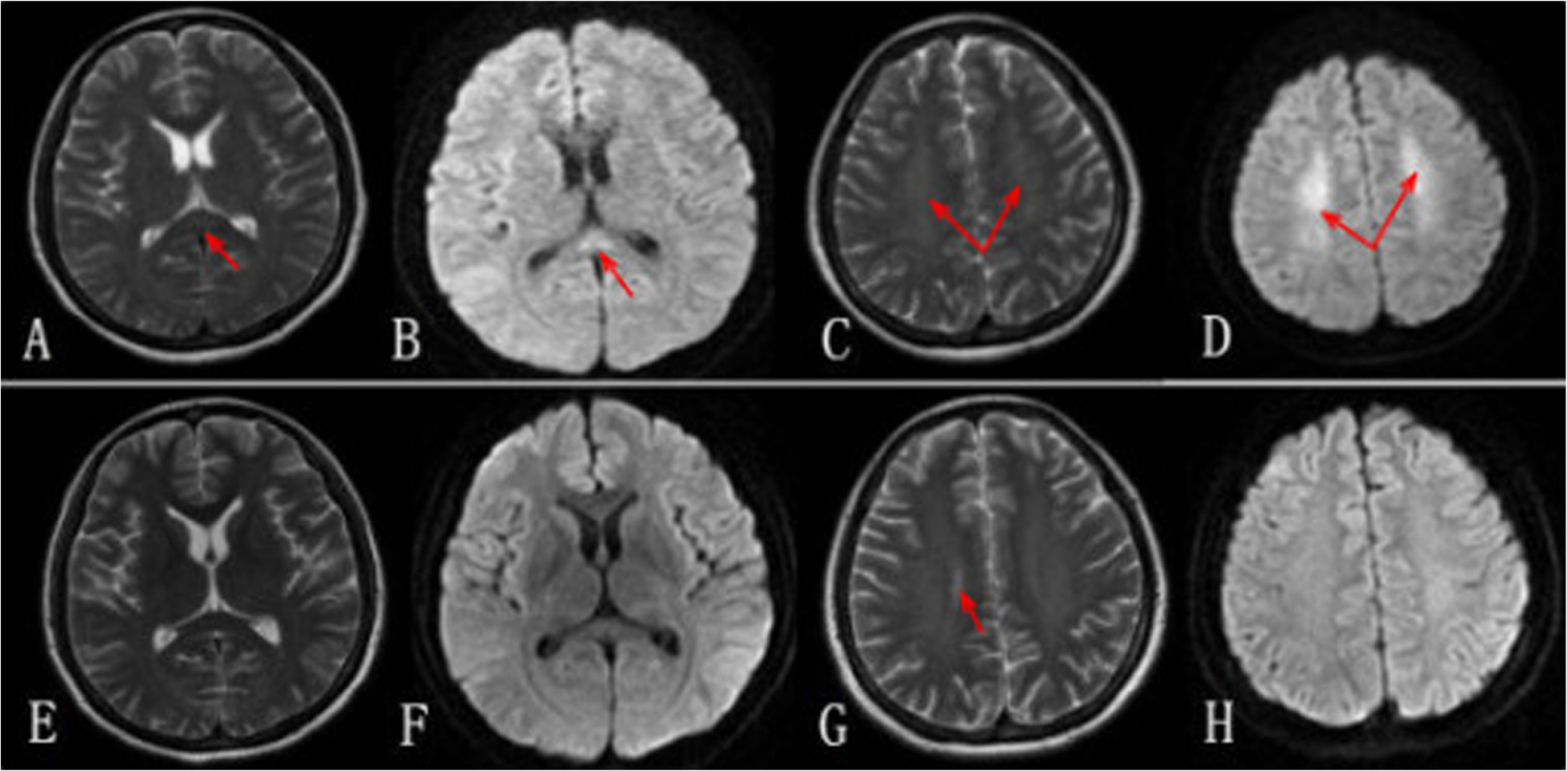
MRI on the day of admission (a, b, c, d) showed a focal high-signal lesion (arrow). After effective treatments (e, f, g, h), the splenial lesion had almost disappeared in the SCC
